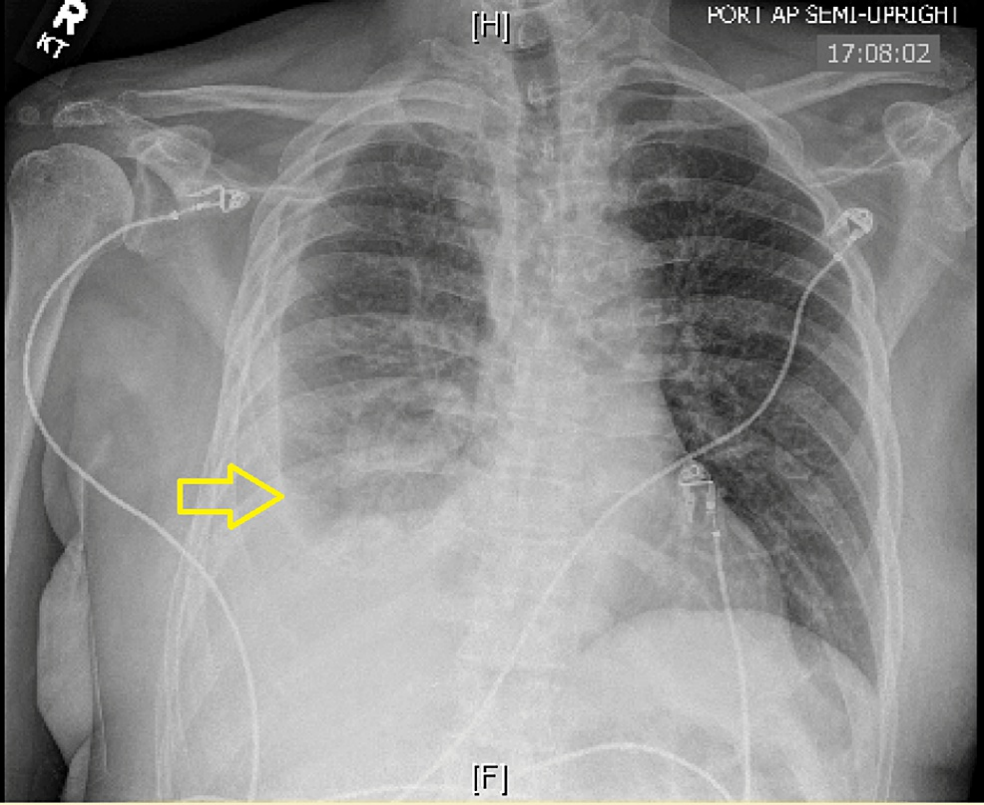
Chest X-Ray The arrow indicates right-sided pleural effusion.
